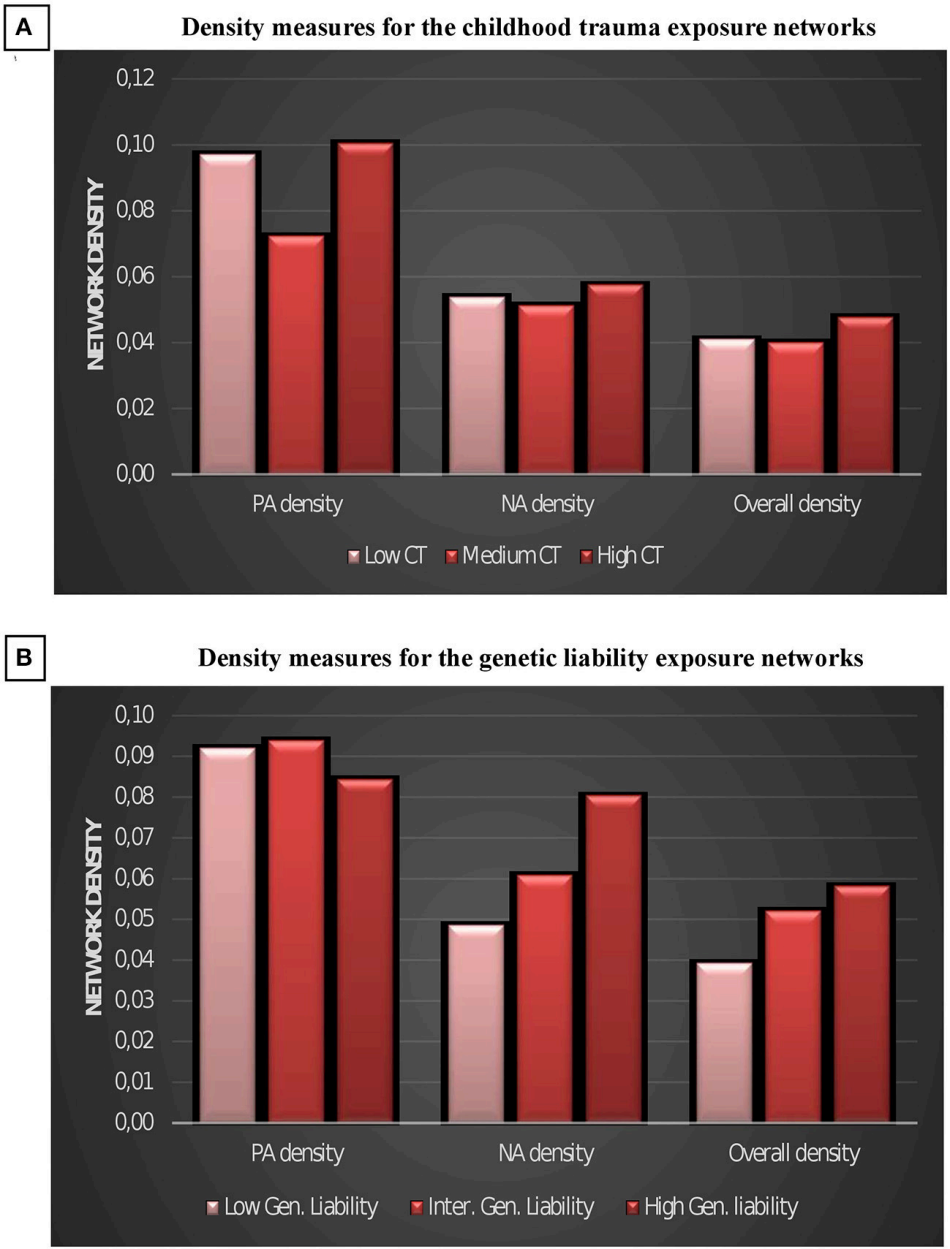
Density measures for the childhood trauma exposure emotions networks (A) and for the genetic liability emotions networks (B). CT, childhood trauma; GL, Genetic liability; NA, negative affect; PA, positive affect.
